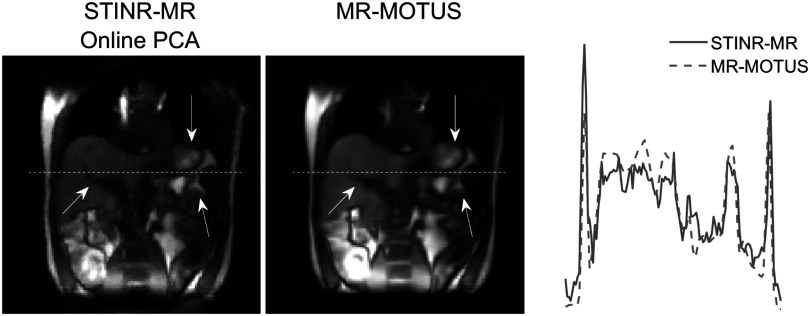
Comparison of reconstructed reference-frame MR images and the corresponding line profiles for the UMCU human subject study. The corresponding location of the line profiles was indicated by the horizontal dashed lines, and the arrows highlighted the over-smoothed regions of MR-MOTUS.
